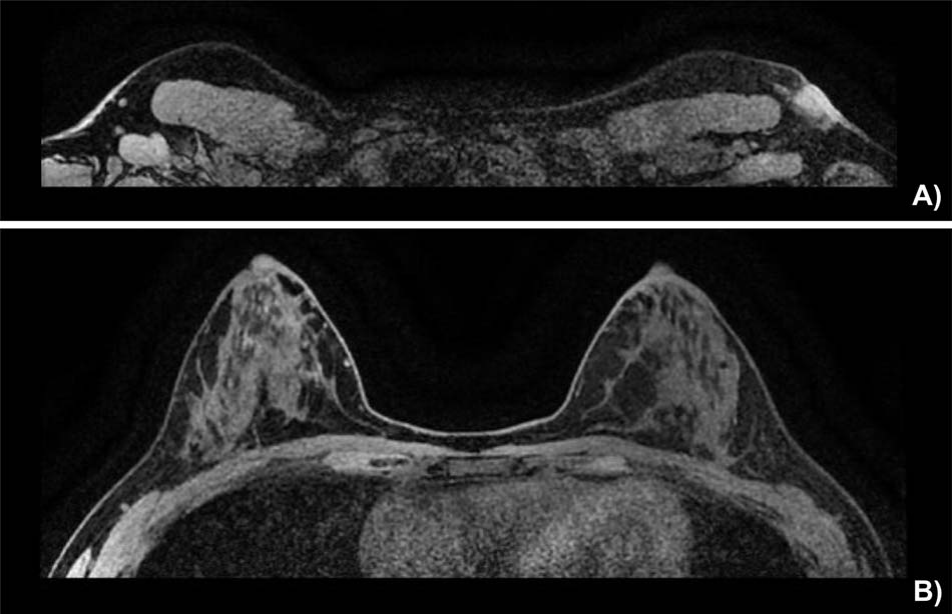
Breast MRI depicting (A) the known mass in a superficial axillary position with direct invasion of the overlying skin and dermal thickening, measured at 1.8 × 1.3 cm wide by 1.9 cm in height. The left axillary nodes appear more numerous and slightly enlarged but share no malignant features. (B) The left and right breast showing no sign of neoplasia.
